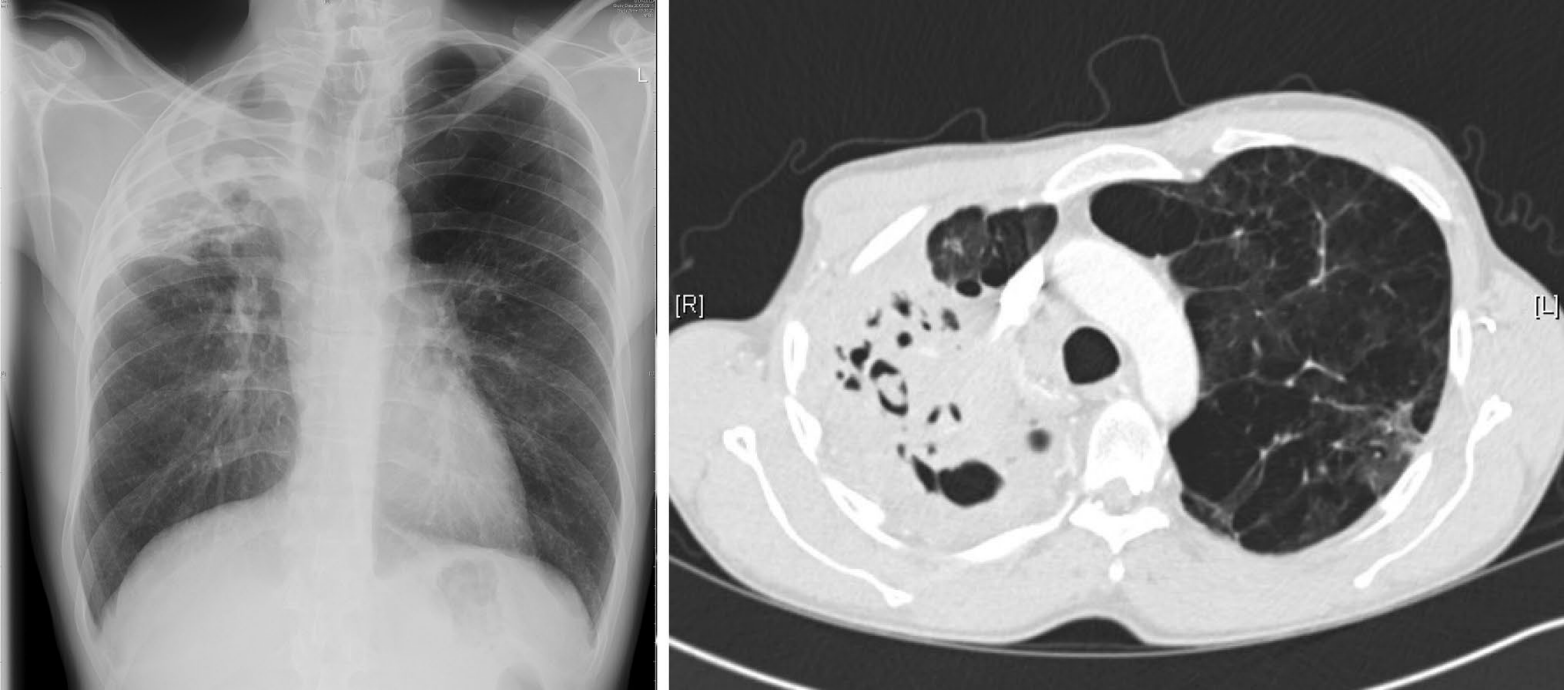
A chest radiograph and CT scan of a patient with COPD showing contraction of the right upper lobe with several large cavities and pleural thickening visible. No fungal balls are seen within the cavities. The left upper lobe is almost completely replaced by emphysematous bullae
